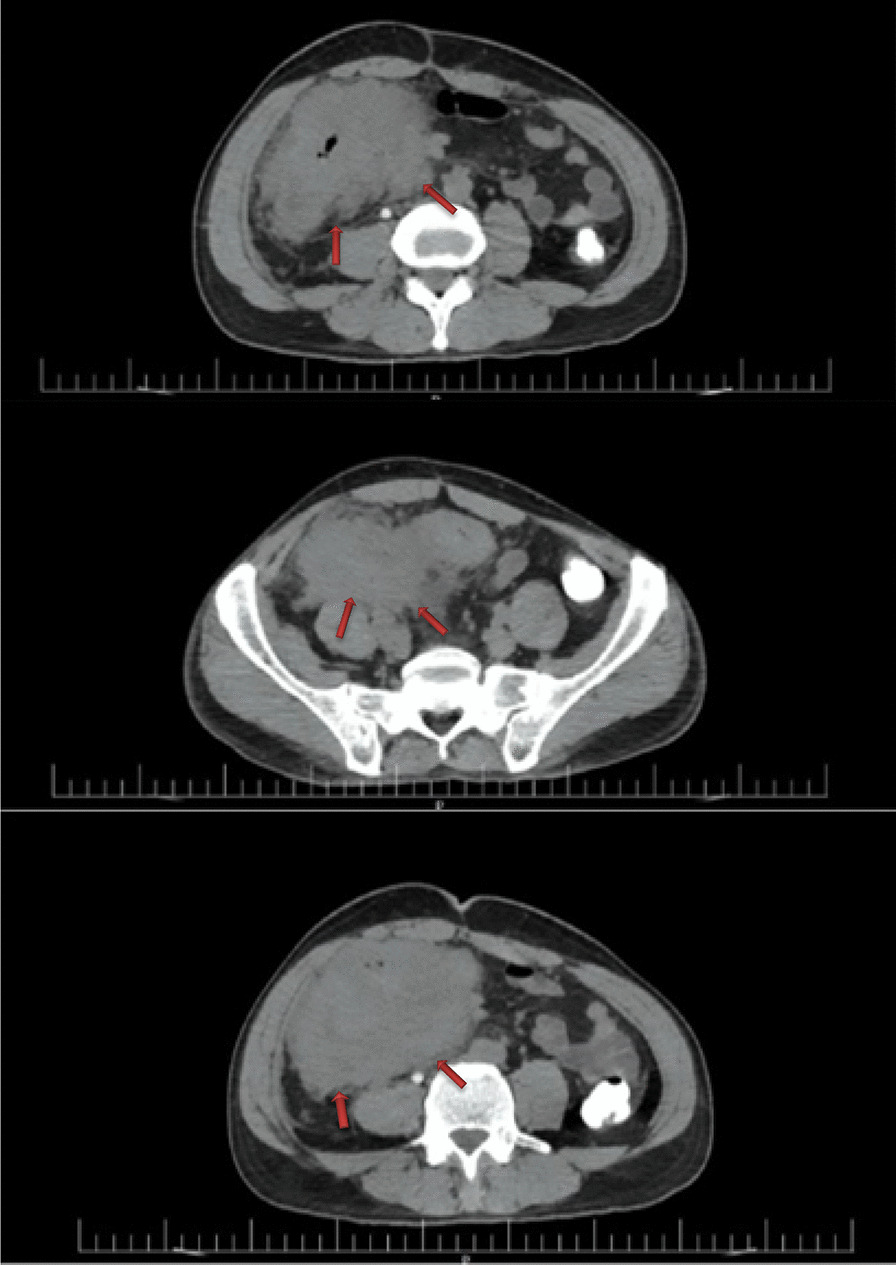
Sections of spiral abdominopelvic CT scan with contrast show a large mass surrounding with fat
